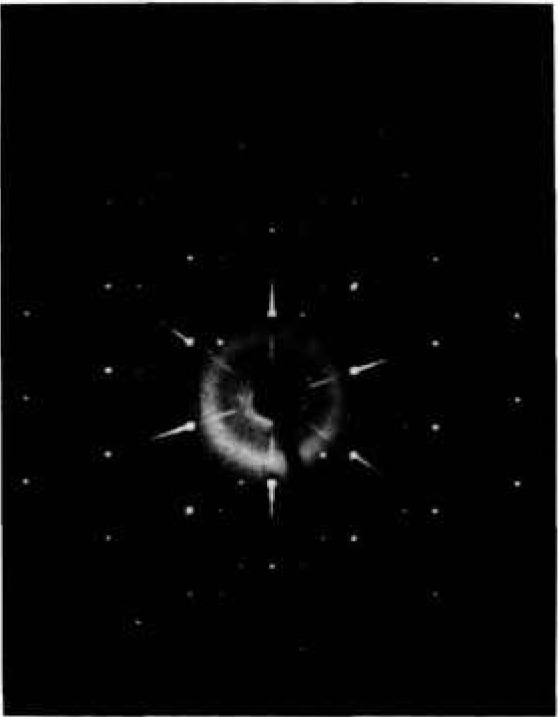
x-ray precession photograph of the fcc α_1_*″* phase (Mo radiation).
